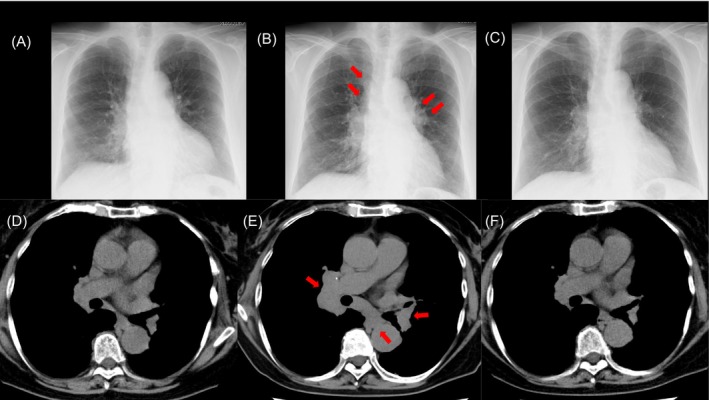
Changes in radiological findings. Chest radiograph findings (A) 4 months before sarcoidosis diagnosis, (B) at sarcoidosis diagnosis and (C) 4 months after sarcoidosis diagnosis. Chest high‐resolution computed tomography findings (D) 6 months before sarcoidosis diagnosis, (E) at sarcoidosis diagnosis, and (F) 6 months after sarcoidosis diagnosis.
